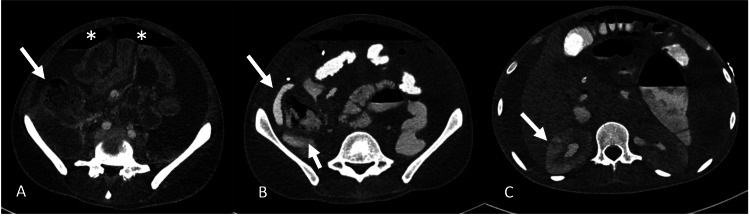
CT abdomen and pelvis at the time of the presentation. (A) Initial late arterial phase sequence without oral contrast showing sluggish to non-enhancement of the cecum (white arrow) with free intraperitoneal air as indicated by (*). (B, C) Repeat scans performed on the same day but a few hours later with oral and no intravenous contrast; (B) Oral contrast extravasation adjacent to the cecum indicating perforation; (C) Delayed contrast excretion from segments of the kidney indicating acute renal impairment.
